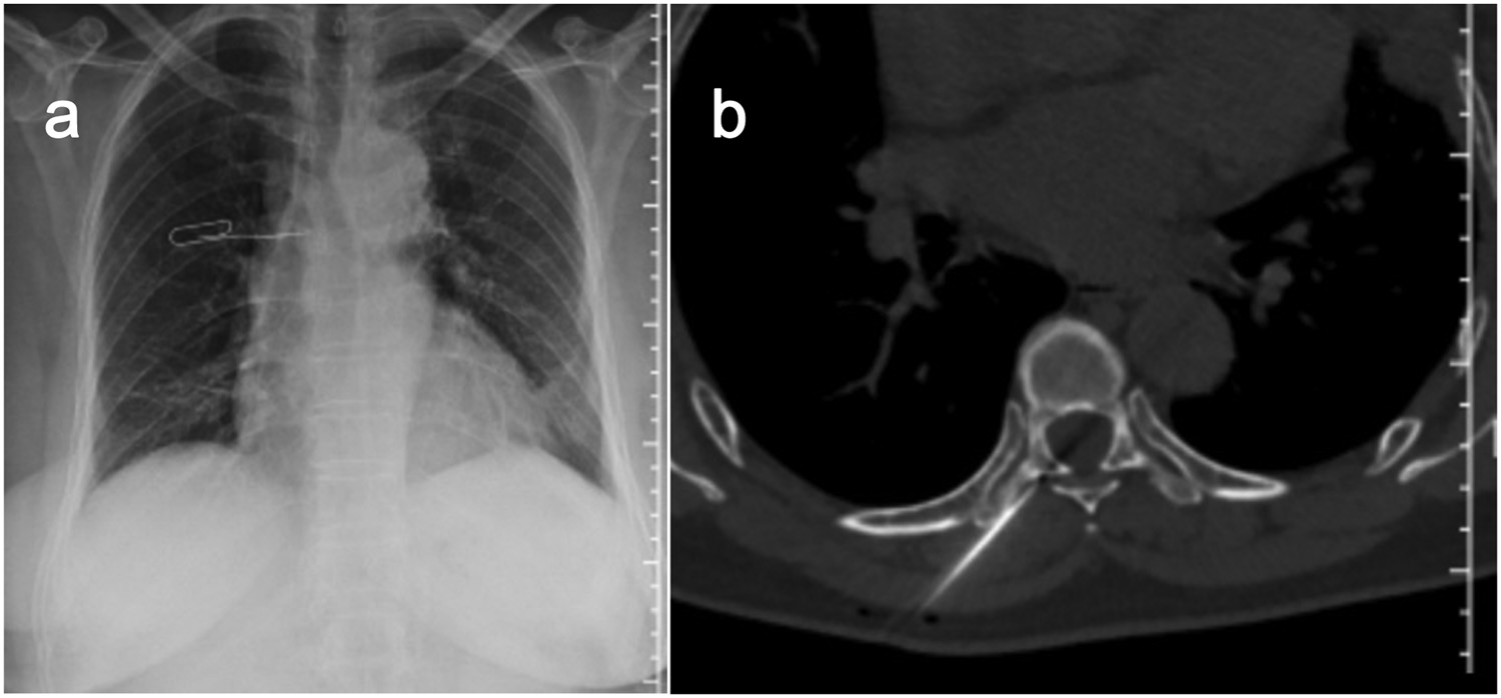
Localization is performed on the body surface by X-ray (a) and puncture is performed under CT guidance (b).
